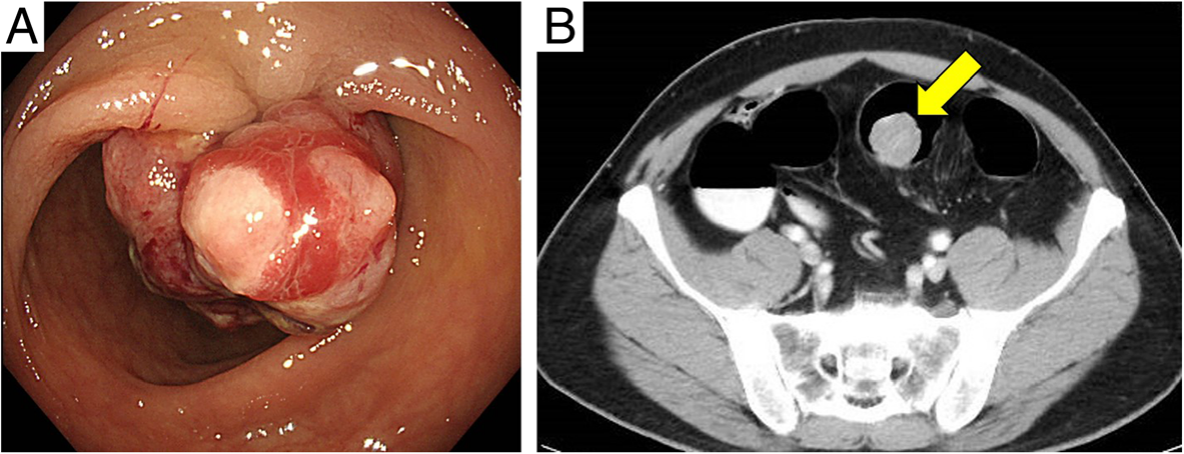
a Endoscopic findings: colonoscopy revealed a large polypoidal tumor in the sigmoid colon. b Contrast-enhanced computed tomography findings: the position of the tumor is indicated; no metastasis to lymph nodes or a distant site was evident
